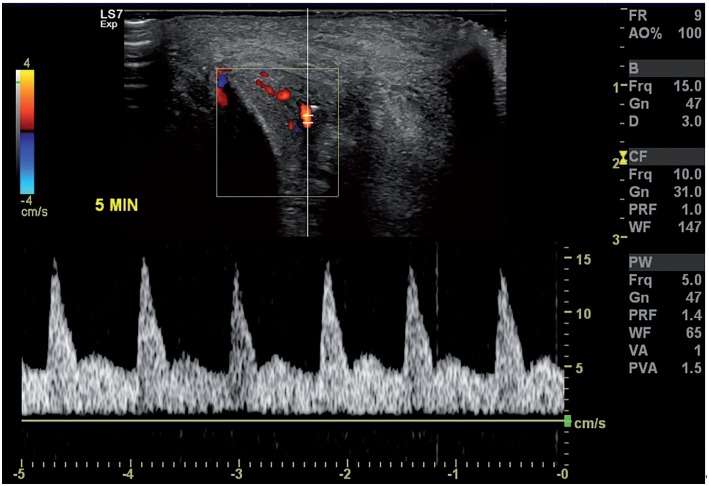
Penile color duplex Doppler ultrasound after pharmacological induced erection test through Alprostadil intracavernous injection excluding vascular disease in a patient with erectile dysfunction after a penile fracture.
